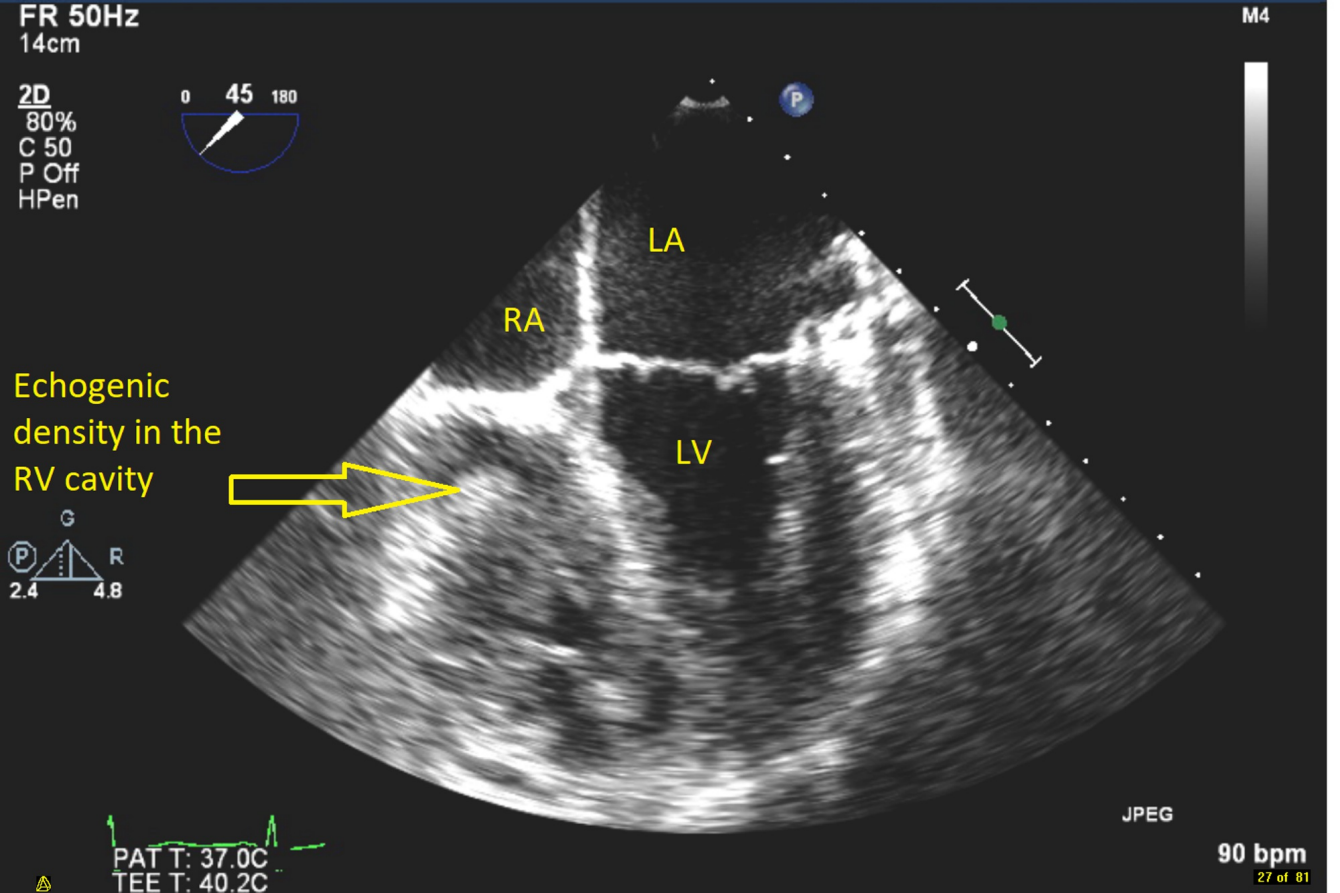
Transesophageal echocardiogram mid esophageal view showing a clearer echogenic density in the cavity of the right ventricle (RV) measuring 31 x 20 mm suggestive of a mass along with right atrium (RA), left ventricle (LV), and left atrium (LA).
